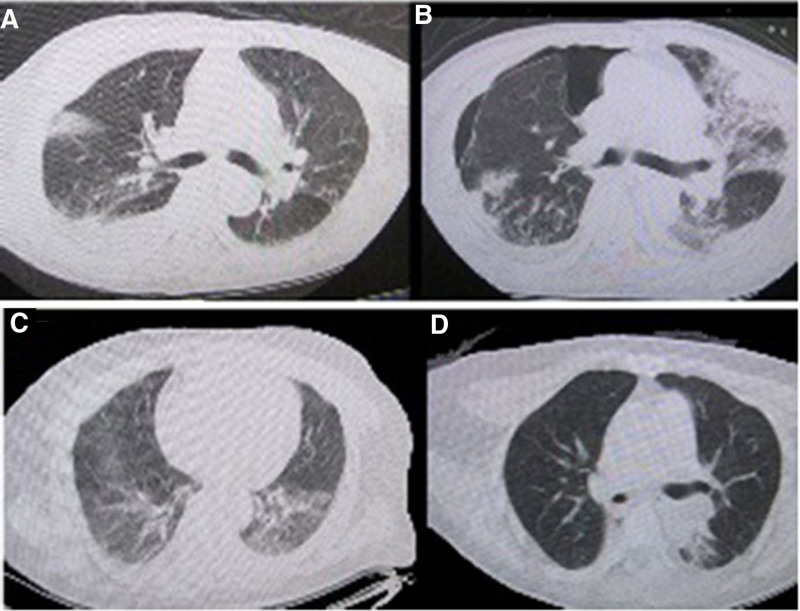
(A and B) is from a patient with cough and dyspnea for 2 days, chest CT on ICU demonstrated that the opacity in the bilateral lungs (A) and Lab tests showed elevated 4 MIM and patient was in respiratory failure requiring mechanical ventilation. on ICU day 16, CT showed pneumonia (B) had not improved, and the patient was diagnosed with SAMI following CAP and died in the ICU. (C and D) is from a patient with bilateral pneumonia on the day after onset, chest CT demonstrated that the bilateral pneumonia (C) Lab tests confirmed elevated 4 MIM. On ICU day 3, patient was in respiratory failure requiring mechanical ventilation and The patient was diagnosed with SAMI. On day 6 days, chest CT showed pneumonia have improved (D) and finally recovery. CAP = community-acquired pneumonia, ICU = intensive care units.
